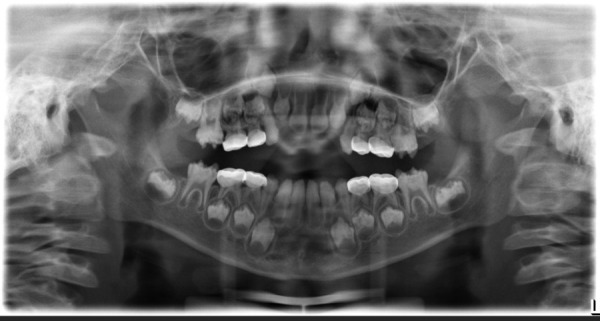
Panoramic radiography showing radiographic signs consistent with amelogenesis imperfecta. Misfitting preformed metal crowns alter the eruption of permanent molars.
